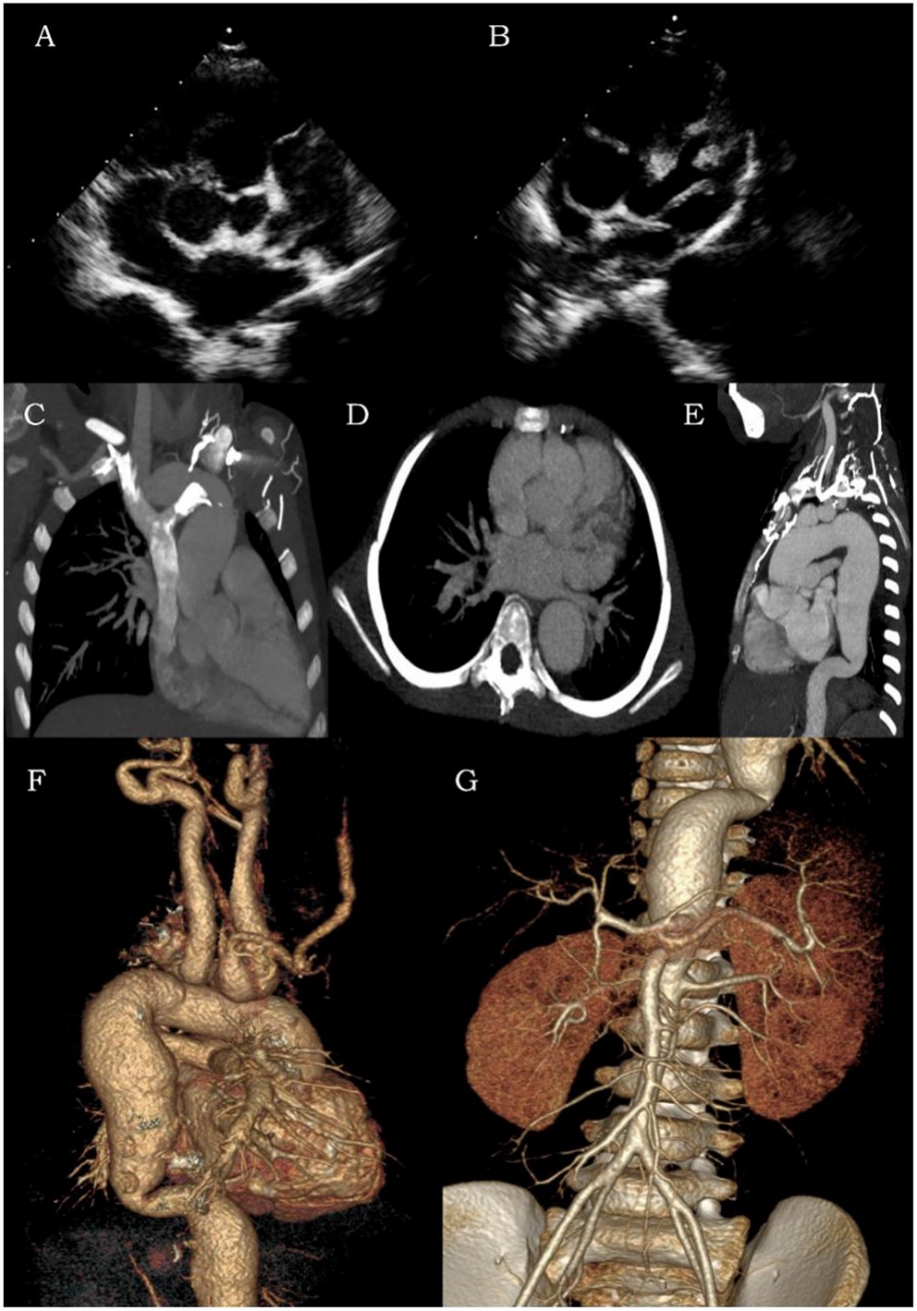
Transthoracic echocardiography (A,B) and computed tomographic angiogram (C–G) of coronary arteries, aorta, and its major branches. The contrast CT and color ultrasound showed multiple areas of arterial dilation in the aorta, coronary artery, bilateral carotid arteries, subclavian artery, brachiocephalic artery, innominate artery, and bilateral axillary arteries as well as compressed and narrowed jugular and left brachiocephalic veins with backflow obstruction.
